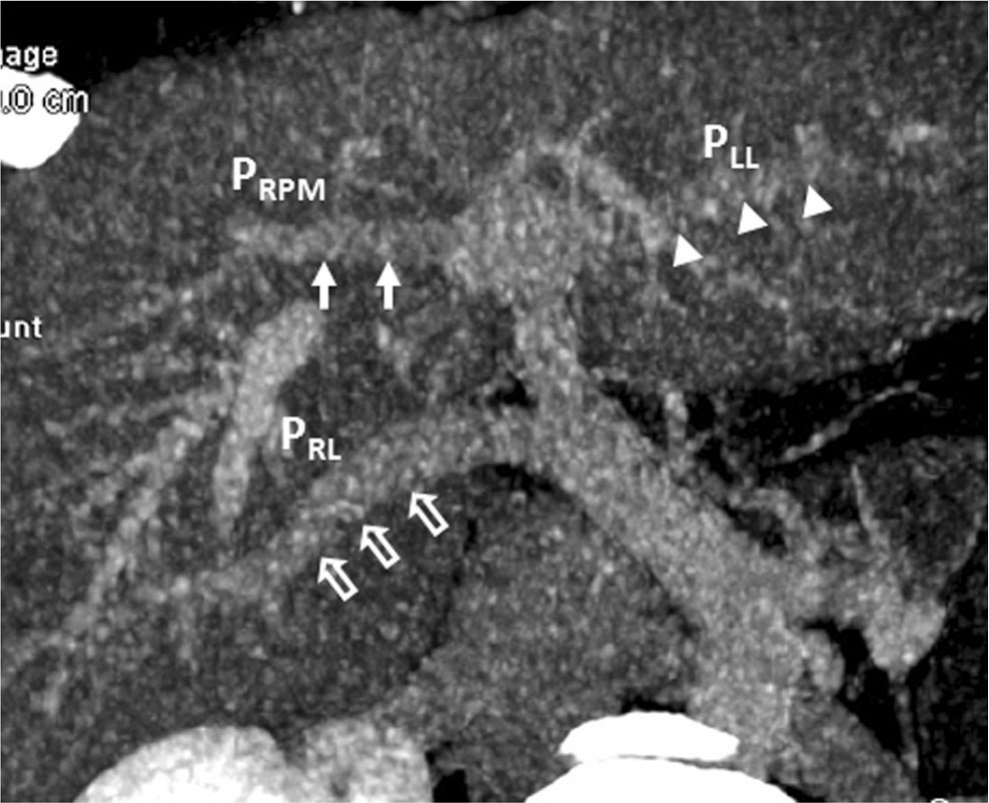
Contrast-enhanced computed tomography (CT) with maximum intensity projection (MIP) reconstruction revealing portal vein ramification of a Shindoh’s independent right lateral type
